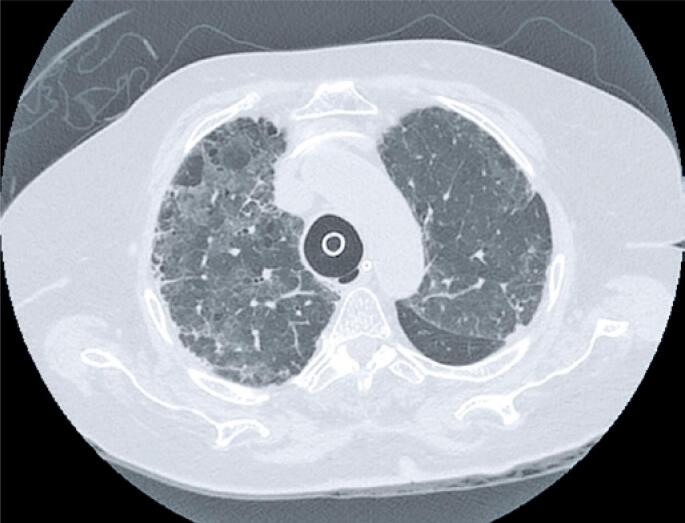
Cross-sectional computed tomography scan of COVID-19 patient showing loss of continuity of the tracheal posterior wall (tracheal tear). Note the formation of a small air sac in the lacerated region
